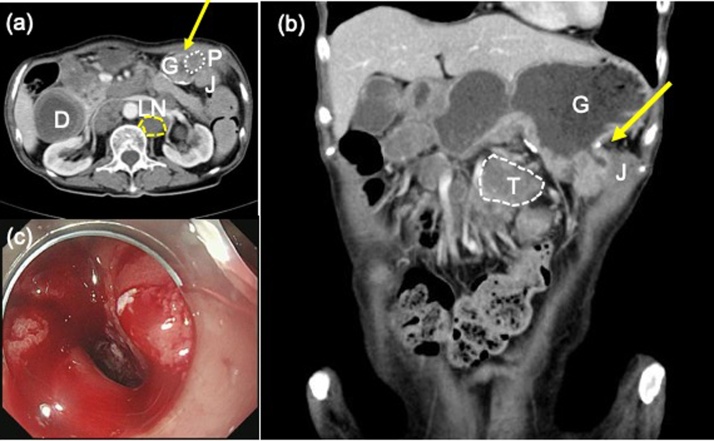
Pre-operative CT, endoscopic findings. (a, b) CT scan reveals stricture of the gastrojejunal anastomosis (yellow arrow) due to peritoneal dissemination (P/white dotted line). The stomach (G) and duodenum (D) are dilated. The primary tumor (T/white dotted line) and lymph node metastasis (LN/yellow dotted line) is also seen. J: jejunum. (c) Endoscopy revealing invasion of the tumor into the lumen of the anastomosis.
